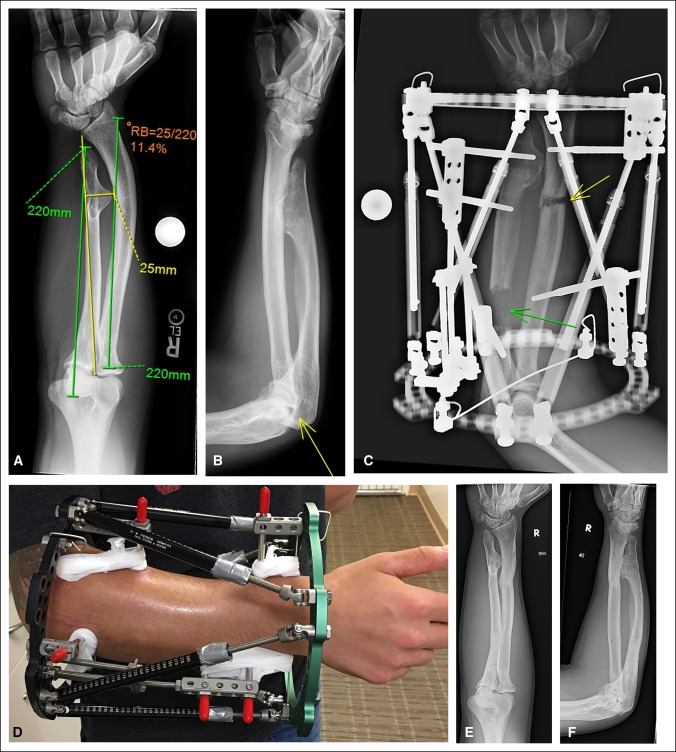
A, Figure demonstrating a patient with multiple hereditary exostosis who presented with gross deformity of forearm. The bone lengths are equal, but the radial bow is increased, leading to ulnar translocation of carpus (measurements originally described by Burgess et al). B, Although the radial head did not dislocate during growth in this patient, subluxation is evident (yellow arrow). C and D, External fixator used to lengthen the ulna (green arrow) and correct the radial deformity (yellow arrow). E and F, Radiographs of forearm after fixator removal demonstrating improved position of carpus and radial head.
